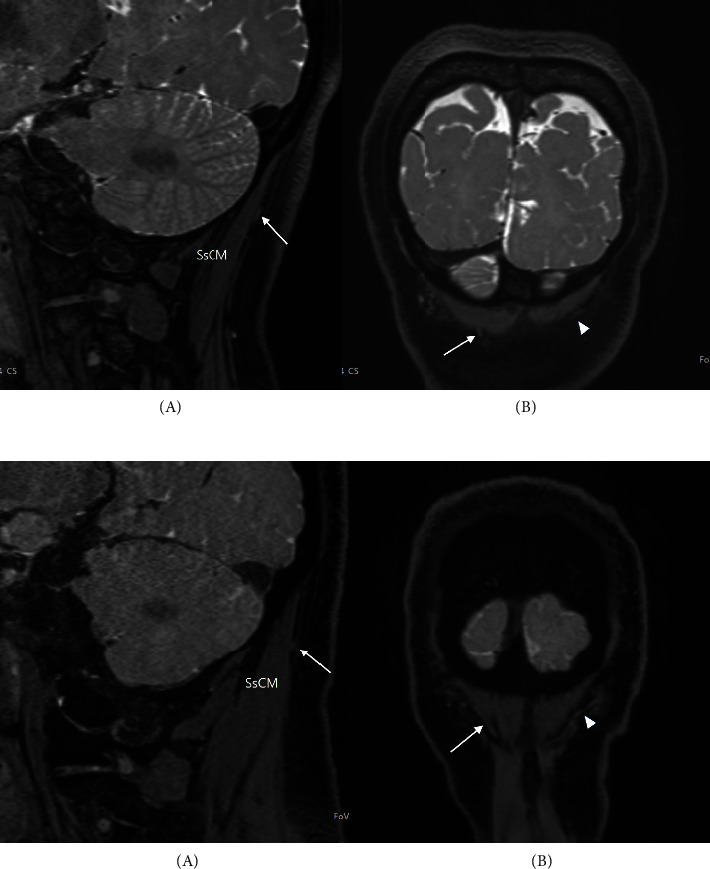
MRI images of the greater occipital nerve (GON). (a) Sagittal (A) and coronal (B) MR images of the right GON (white arrow) in the current case. The left image represents a sagittal T2-weighted image (t2_de3d_we,_iso 0.7 mm, MPR, Magnetom Terra, Siemens, Germany) of the right GON taken along the distal portion path within the trapezial tunnel. The image on the right is a coronal MR image (t2_de3d_we, iso 0.7 mm, MPR) showing the course of the right and left (white arrowhead) GONs within the trapezial tunnel. SsCM; semispinalis capitis muscle. (b) Sagittal (A) and coronal (B) MR images of the right GON (white arrow) taken with the same MR sequence (t2_de3d_we,_iso 0.7 mm, MPR) in a patient suffering from right trigeminal neuralgia. This is presented for comparison with images from patients with GON entrapment. The image on the right is a coronal MR image (t2_de3d_we, iso 0.7 mm, MPR) showing the course of the right and left (white arrowhead) GONs within the trapezial tunnel. This MRI image shows that it is still difficult to clearly diagnose GON entrapment even with high-resolution MRI. SsCM, semispinalis capitis muscle.
